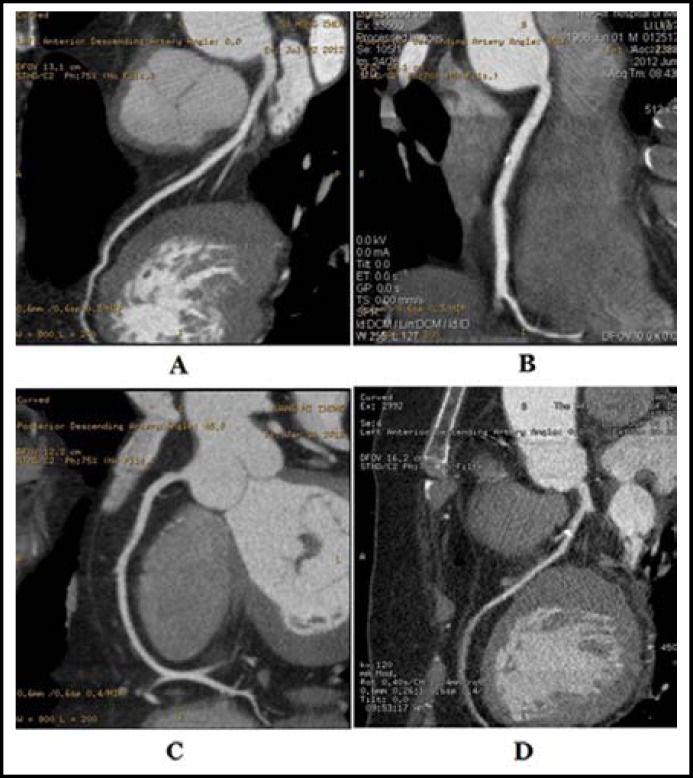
64-slice spiral CT coronary angiography images of the 4 plaque types. A: soft plaque of LMA; B: mixed plaque of middle RCA; C: fibrous plaque of proximal and middle RCA; D: calcified plaque of proximal LAD
